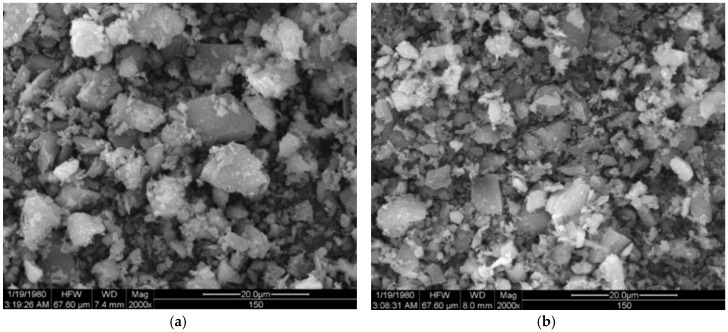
Particle morphology of CT after being ground for (a) 30 min; and (b) 60 min.
